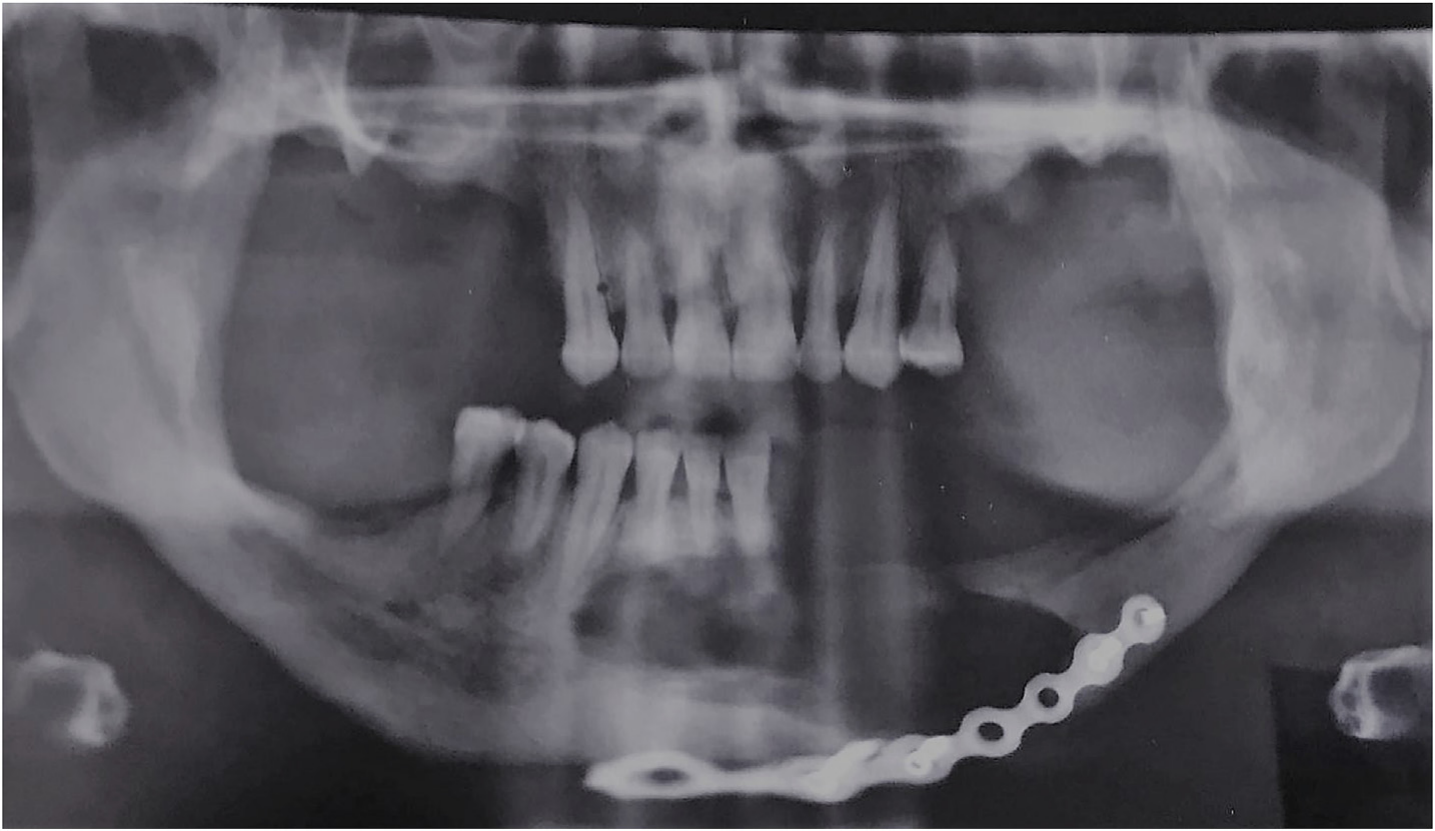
Post-operative orthopantomogram showing the good reduction of the mandibular fracture after bone osteosynthesis with a miniplate.
